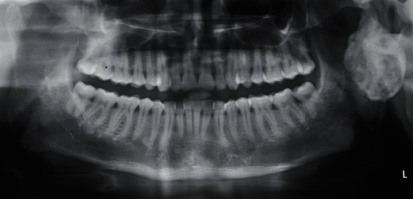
Panoramic radiograph view showed a well-defined radiopacity located at the left posterior ramus of the mandible region.
